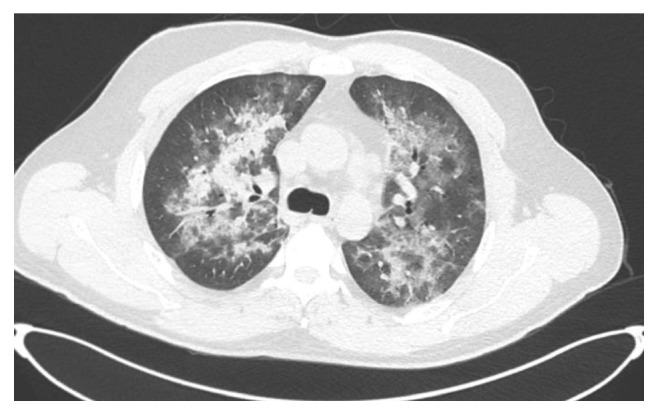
A chest-computed tomography scan revealing areas of parenchymal consolidation and diffuse ground-glass opacities.
